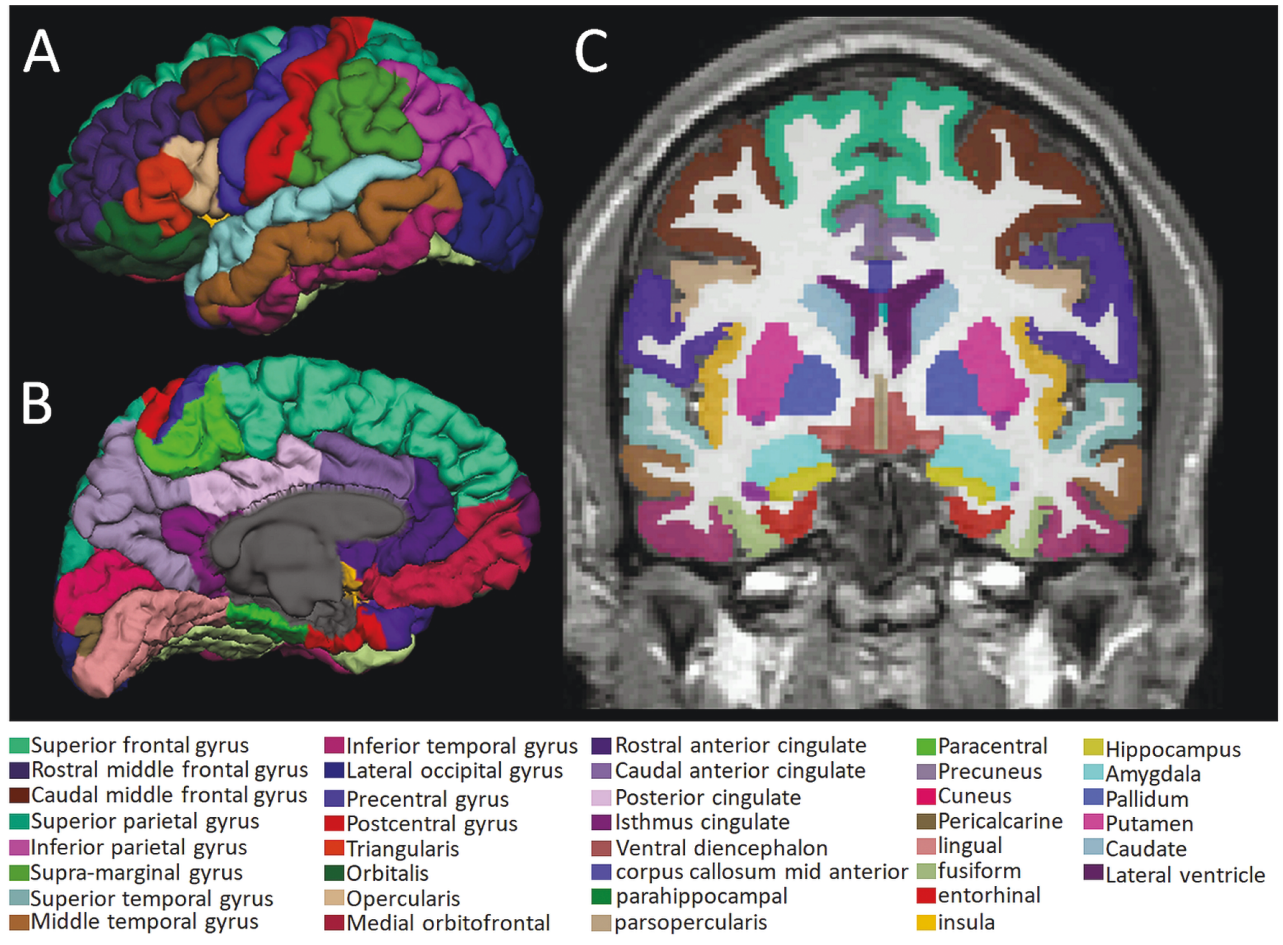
Visualization of the cortical and subcortical segmentation of T1-weighted anatomical data from a representative patient. The figure shows cortical parcellations (external surface) of the three-dimensional brain-extracted data (A and B) and segmentation of the cortical and subcortical structures (internal surface) overlaid on a coronal slice of the raw data (C). Each structure is labeled with a unique color distinction.
